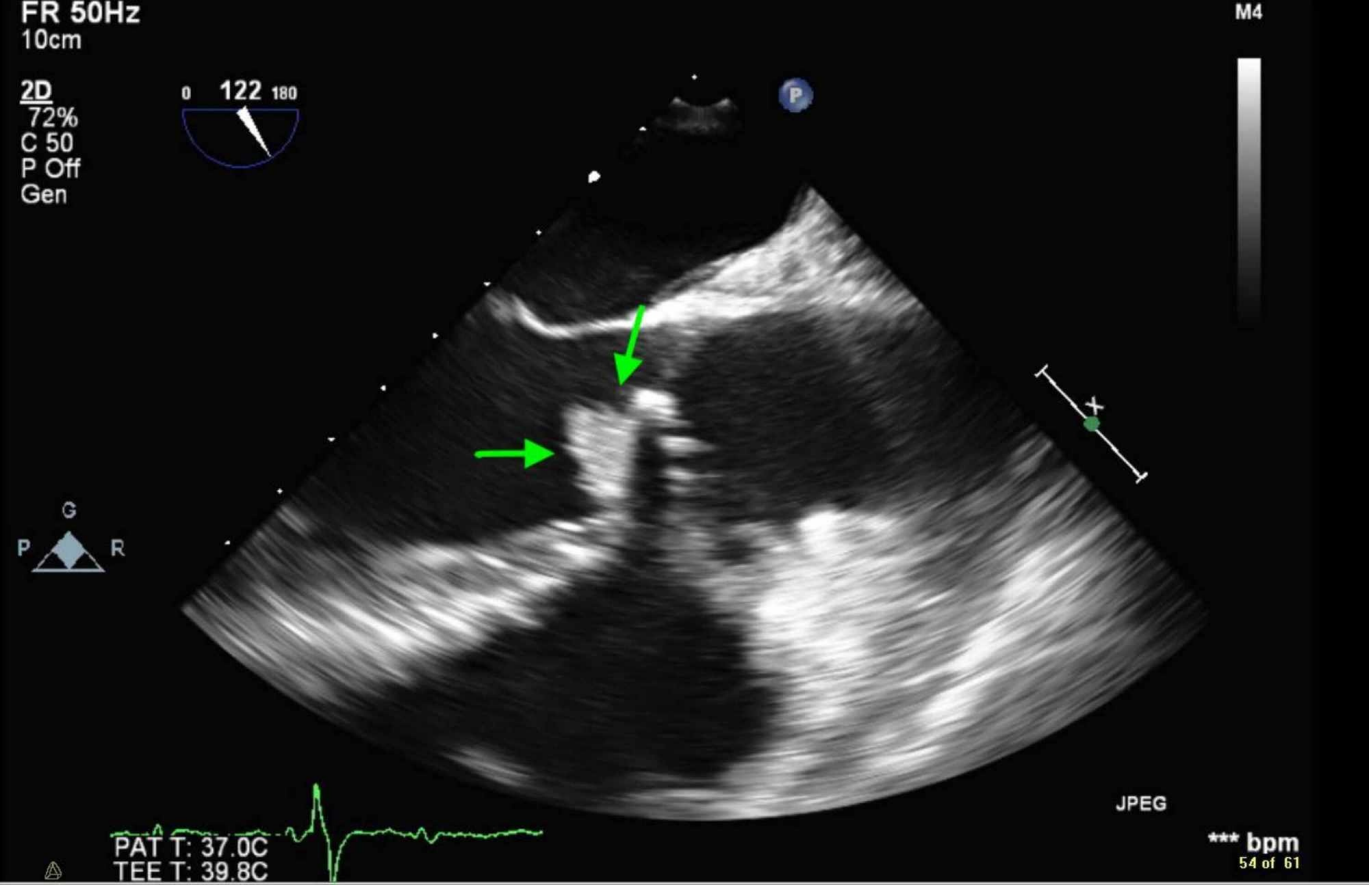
Transesophageal echocardiogram showing a 9x9 mm echodensity attached to the ventricular side of the aortic valve, which represents vegetation
